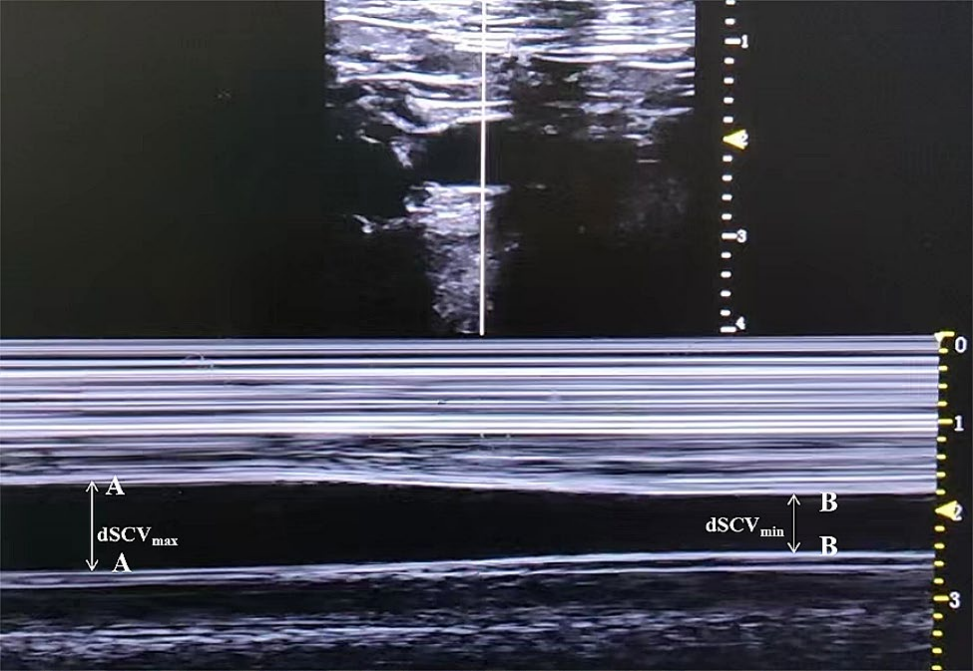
M-mode ultrasonography of the subclavian vein
